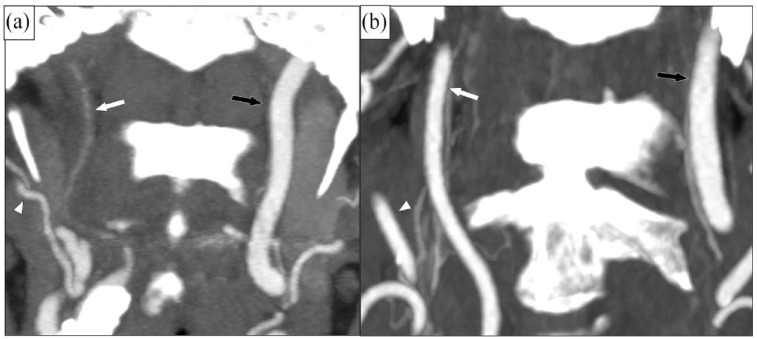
Two cases of right-sided near-occlusion. Coronal CTA. (a) Near-occlusion with full collapse. Beyond a severe stenosis (not in plane), the distal ICA (white arrow) has a threadlike appearance (approximately 0.7 mm diameter), clearly smaller than contralateral distal ICA (black arrow, 4.5 mm diameter) and ipsilateral ECA (white arrowhead, 3.0 mm diameter). ICA ratio 0.16, ECA-ratio 0.23. On PCI-MRI: Ipsilateral ICA flow not detectable, contralateral ICA 330 ml/min, ICA-CBF ratio 0. (b) Near-occlusion without full collapse. Beyond a severe stenosis (not in plane), the distal ICA (white arrow, 2.8 mm diameter) is normal-appearing but smaller than contralateral distal ICA (black arrow, 4.5 mm diameter) and similar to ipsilateral ECA (white arrowhead, 2.1 mm diameter). ICA ratio 0.62, ECA-ratio 1.33. On PC-MRI: Ipsilateral ICA flow 37 ml/min, contralateral ICA 330 ml/min, ICA-CBF ratio 0.076.
